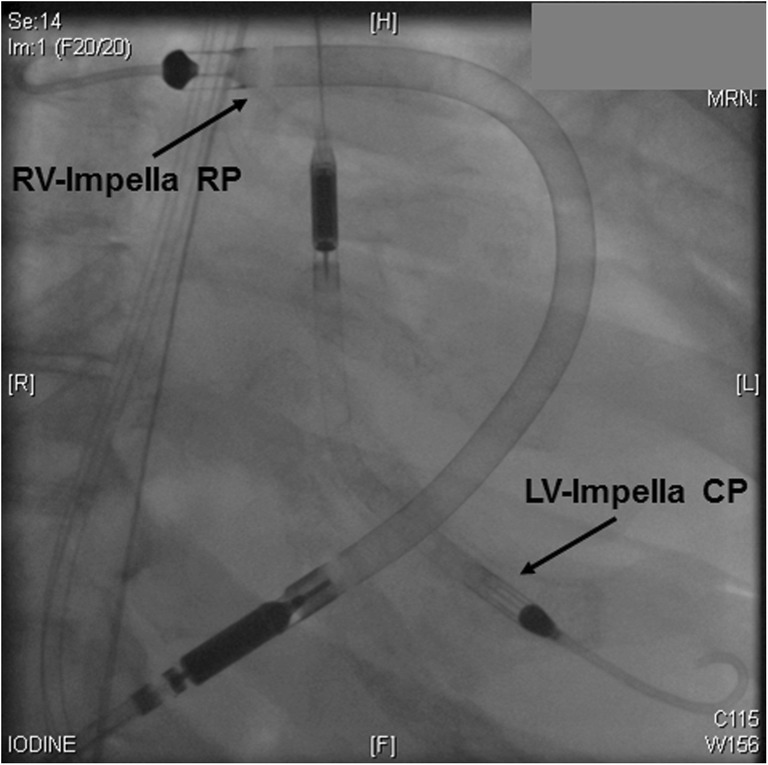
Chest X-ray of a BI-PELLA approach of a patient from our clinic with endomyocardial biopsy-proven severe fulminant myocarditis and biventricular decompensation. RV-Impella RP implanted into the right A. pulmonalis; LV-Impella CP implanted into the left ventricle
